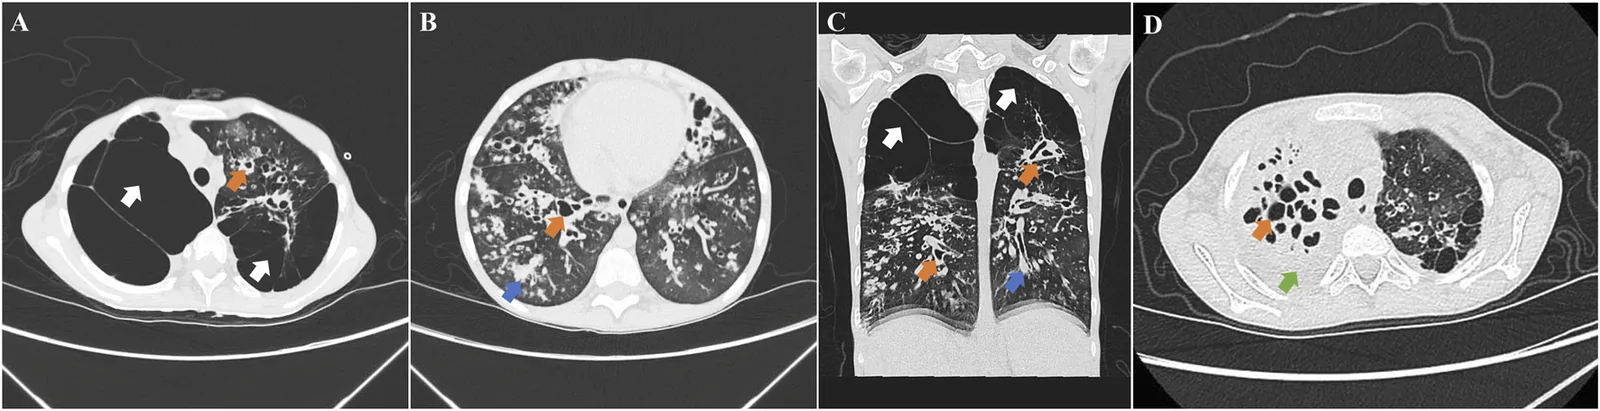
Chest computed tomography of two siblings with end-stage cystic fibrosis. White arrows indicate giant bullae; orange arrows indicate bronchiectasis; blue arrows indicate mucus plugging; green arrows indicate consolidation (A) Proband (16-year-old boy): axial image at the level of the upper lobes showing giant bullae in both upper lobes with near-complete parenchymal destruction (B) Proband: axial image at the level of the lower lobes demonstrating bilateral diffuse bronchiectasis, mucus plugging (C) Proband: coronal reconstruction showing the full craniocaudal extent of lung destruction, with upper-lobe bullous changes and lower-lobe bronchiectasis (D) Younger sister (11-year-old girl): axial image showing extensive consolidation of the right upper lobe with cystic bronchiectasis within the consolidated parenchyma.
